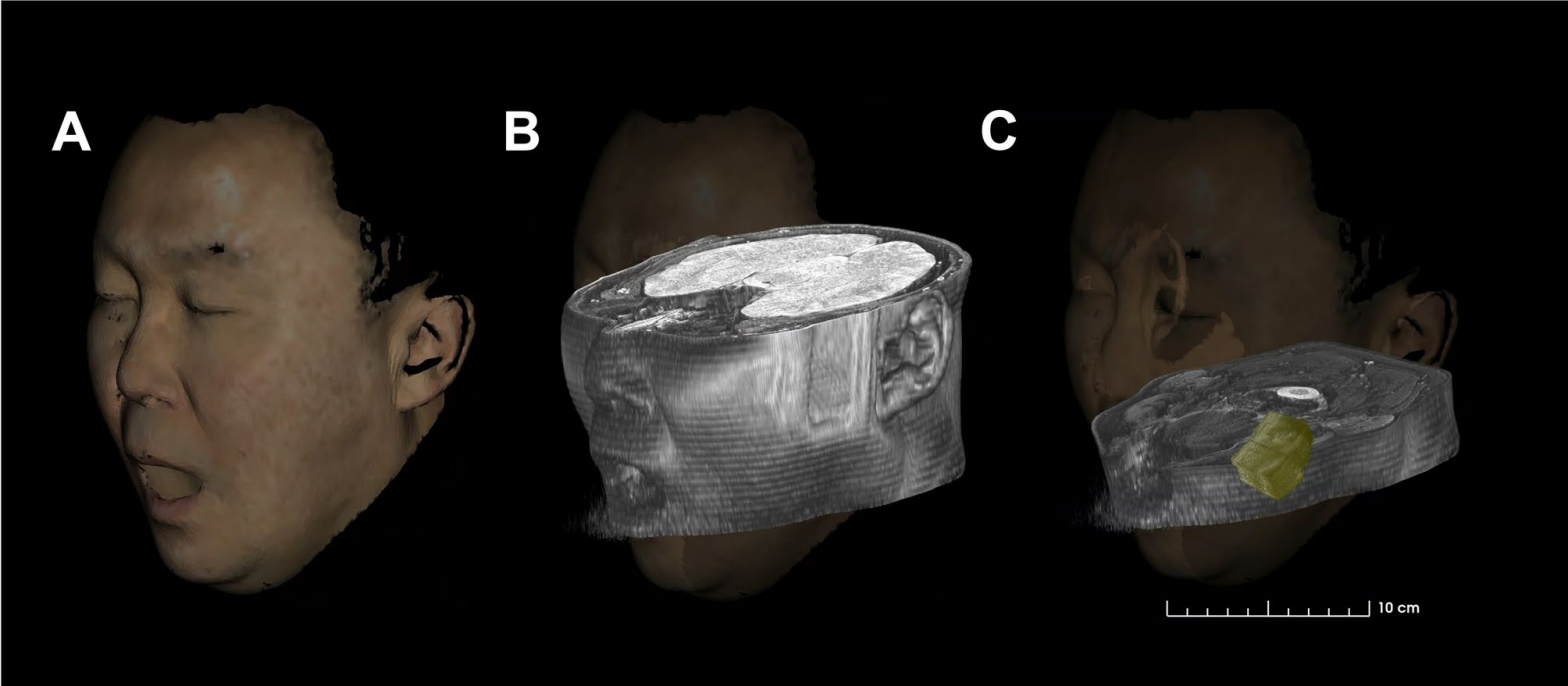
Superimposition process of the three images. Three-dimensional (3D) scanned face (a), combined 3D face scan and magnetic resonance imaging with aligned nose and ear (b), aligned image with tracked intraoral ultrasonography images at the reference point level (c).
